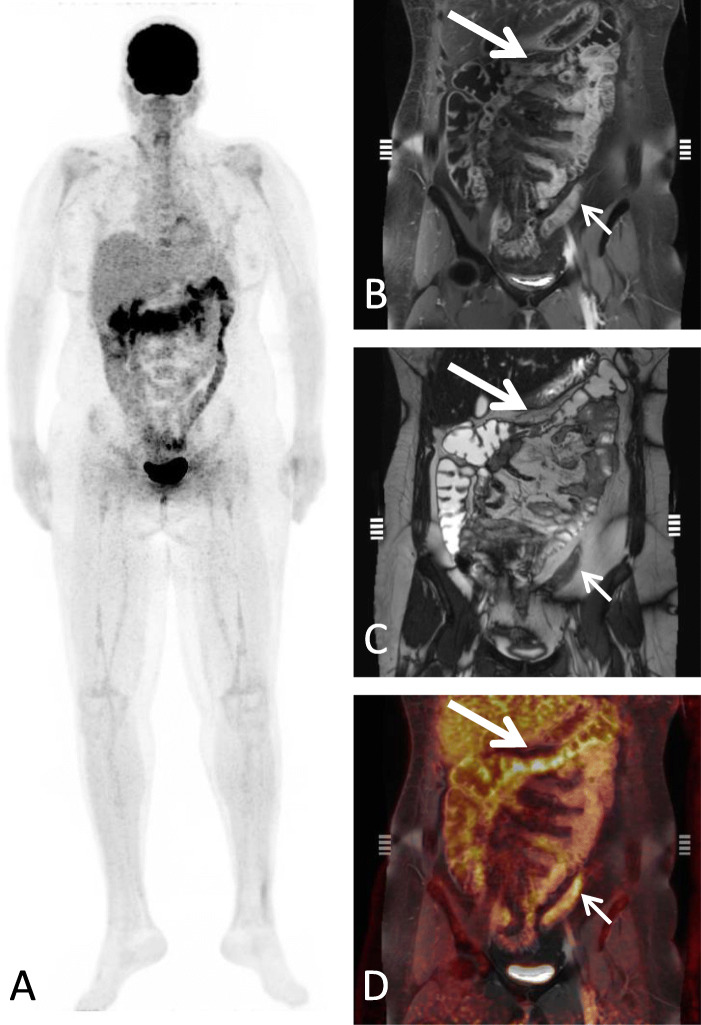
Whole body MIP (A) of an acute intestinal GvHD patient with increased ^18^F-FDG uptake of the colon transversum and descendens. Corresponding coronal MR images reveal mural contrast enhancement of affected large bowel segments (big and small arrow) on T1-weighted contrast enhanced images (B) and stenosis (big arrow) with prestenotic distension as shown on coronal T2-weighted imaging (C) with corresponding increased ^18^F-FDG uptake in fused images (D)
